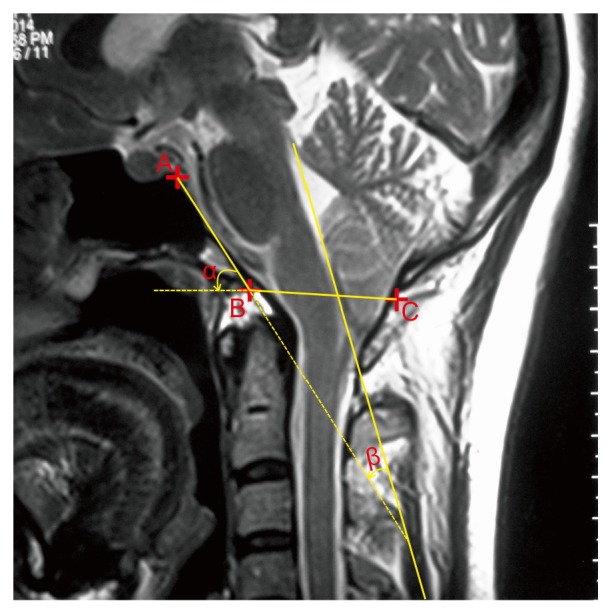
The angle measurements on the median sagittal MRI scan.
